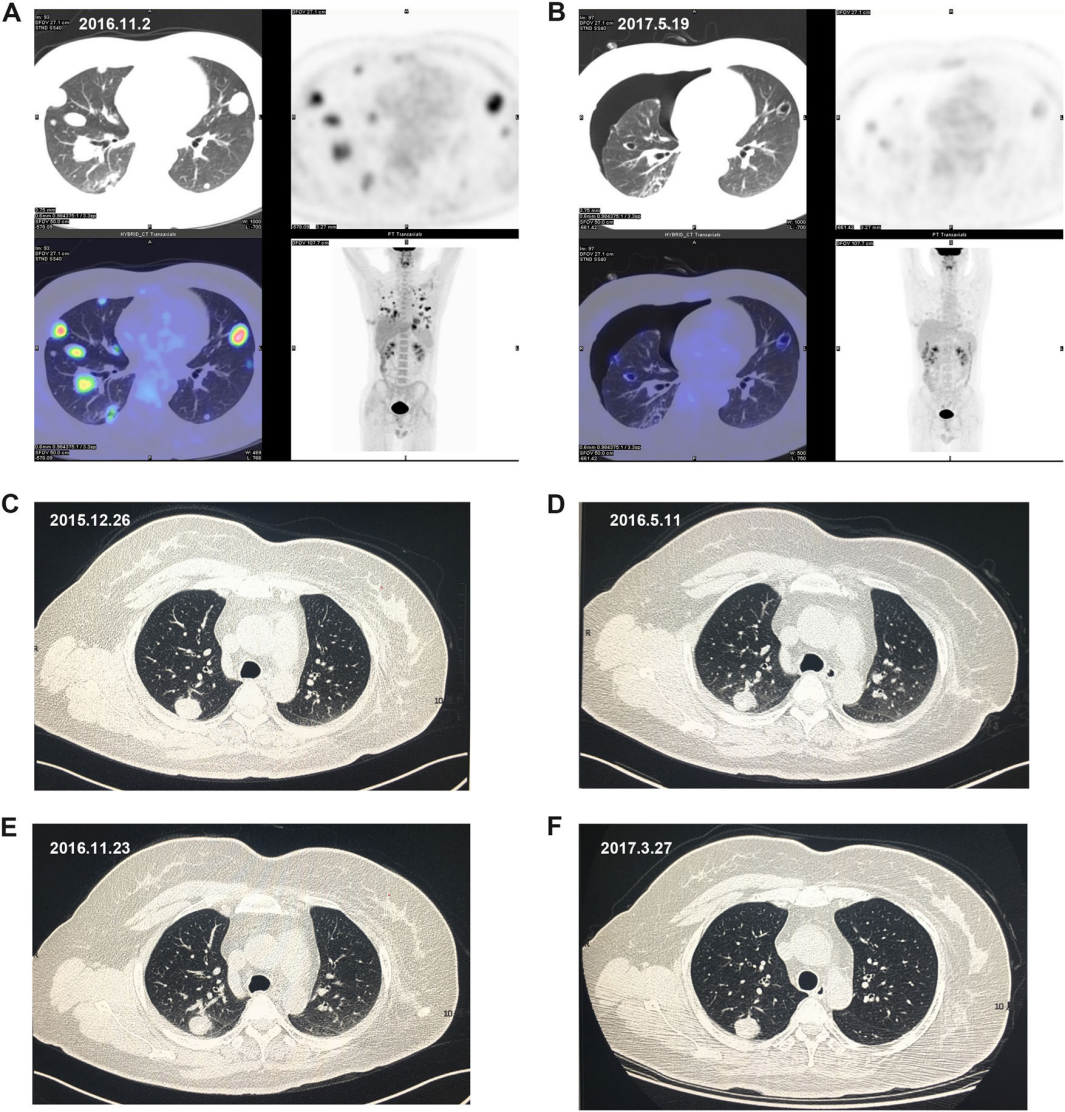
Typical responses to apatinib treatment in two sarcoma patients. a, b Metastatic undifferentiated pleomorphic sarcoma lesions showed significant decrease in size and pulmonary cavities after apatinib treatment. Positron emission tomography-computed tomography (PET-CT) showed decreased tumor size, metabolic activity, pulmonary cavities, and severe pneumothorax after apatinib treatment. a Before apatinib treatment (2016.11.2). b After apatinib treatment (2017.5.19). c–f Stable lung metastatic lesion in patient with synovial sarcoma treated with apatinib. Chest CT scan showed long-term stable disease. c: CT scan before treatment (2015.12.26). d CT scan after treatment (2016.5.11). e CT scan after treatment (2016.11.23). f CT scan after treatment (2017.3.27)
